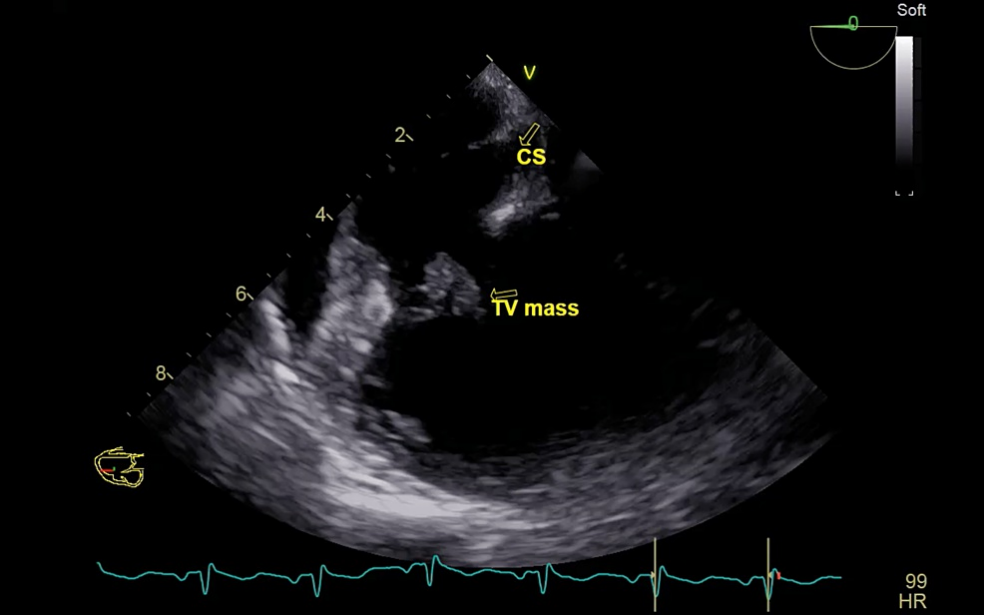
A lower esophageal view at 0 degree of the first transesophageal echocardiogram showing coronary sinus (CS) and a large mass attached to posterior leaflet of the tricuspid valve (TV).
